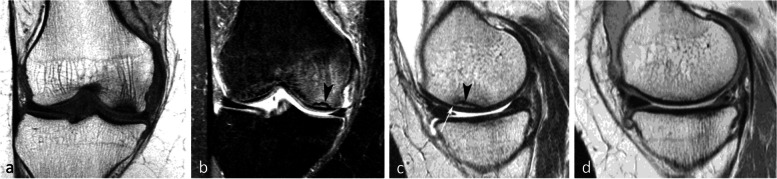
Visibility of subchondral insufficiency fracture (SIF) in a BME-like area. a–c On T1-weighted MR images (a), the fracture can be masked by the neighboring low signal intensity, while on fat-suppressed T2-weighted (b) or T2-weighted images (c) the fracture is apparent (arrowheads), contrasting with the surrounding high signal. In this case of SIF, BME-like high signal intensity is present on both sides of the fracture, including the area between the latter and the articular surface, corresponding to the area with infiltrated but a not degraded fatty marrow. Note that the fracture line does not completely isolate this subchondral area. The potential for this SIF to heal was confirmed by a follow-up study a few months later showing normalization (d)
